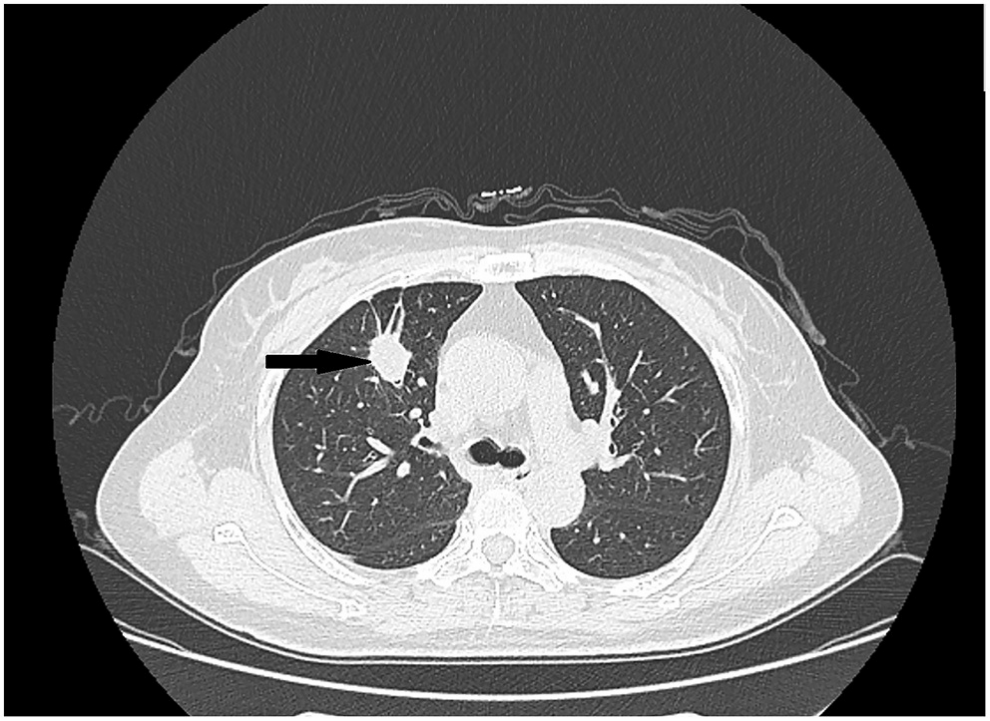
Computed tomography scan showing the primary lung tumor (arrow).
